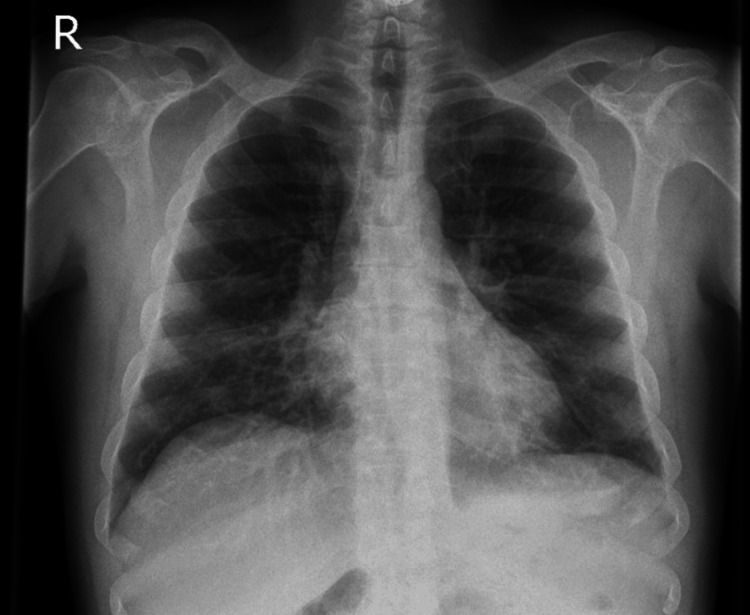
Chest X-ray showing almost complete resolution of previous changes.
